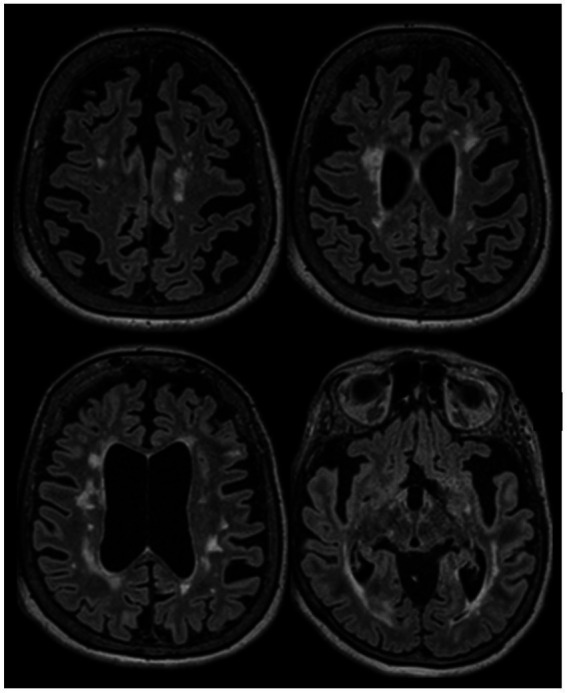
A 66-year-old woman was evaluated to establish care in our clinic. She was diagnosed with RRMS in 2002 after an episode compatible with sub-acute sensory myelitis. She was a participant in a Natalizumab trial, and was treated with this drug between 2003 and 2006. She experienced a relapse in 2007 after Natalizumab discontinuation, and was started on interferon beta1-a IM once a week between 2007 and 2015. She had a pseudo-relapse in 2014 in the context of acute illness. She did not report subacute symptoms since 2014, however she has noticed progressively worsening gait difficulties over the past few years, which she attributes to invalidating mechanical lower back pain related to severe spinal stenosis and degenerative disk disease. She continues to have flu-like symptoms after each interferon injection which last 12 to 24 hours. Her brain MRI in 2022 (at the age of 65) is stable compared to her MRIs between 2015 and 2019, except for a mild worsening of whole brain atrophy. After explaining the risks and benefits of treatment discontinuation, we decided to discontinue her interferon treatment. She has been clinically stable since. *This case highlights that treatment discontinuation is a reasonable option in older patients with long standing disease duration and no clinical/radiological activity*. Axial FLAIR sequences of brain MRI in July 2022 showing extensive lesions in the supratentorial regions which were stable in number and size compared to prior MRI in 2017, associated with diffuse brain atrophy.
